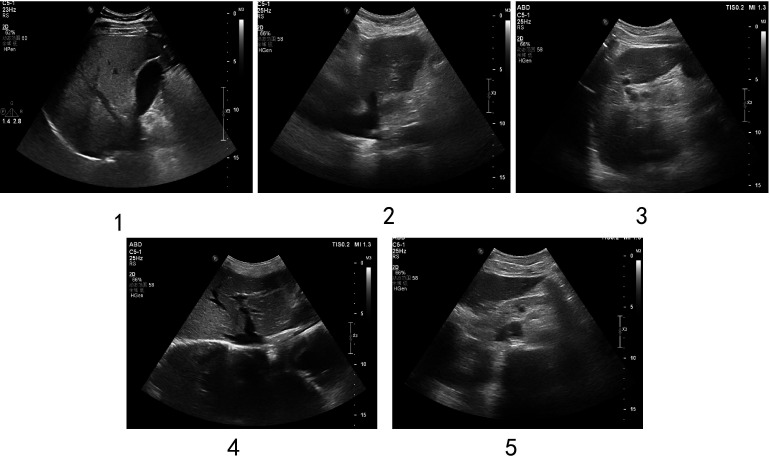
Ultrasound examination revealed an uneven distribution of intrahepatic echoes with thickening and enhanced echogenicity in five abnormal OBI donors.
